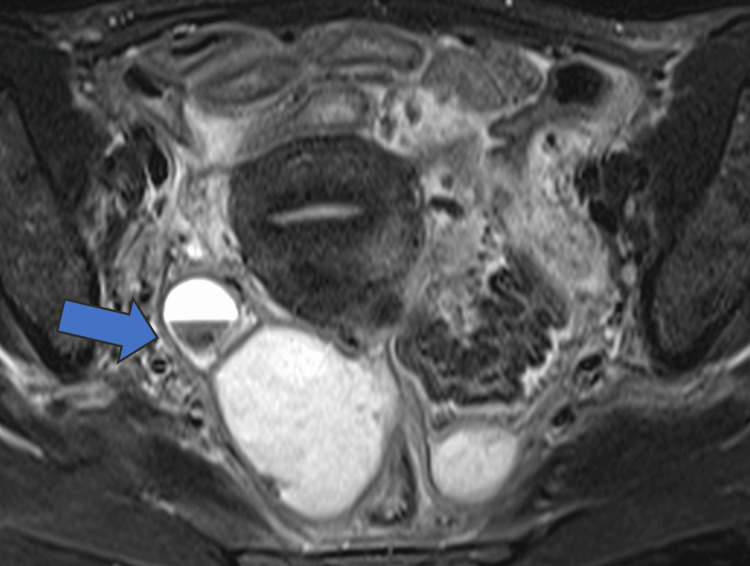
Gadolinium contrast-enhanced MRI scan (horizontal section) Right fallopian tube mass edema (arrow).
